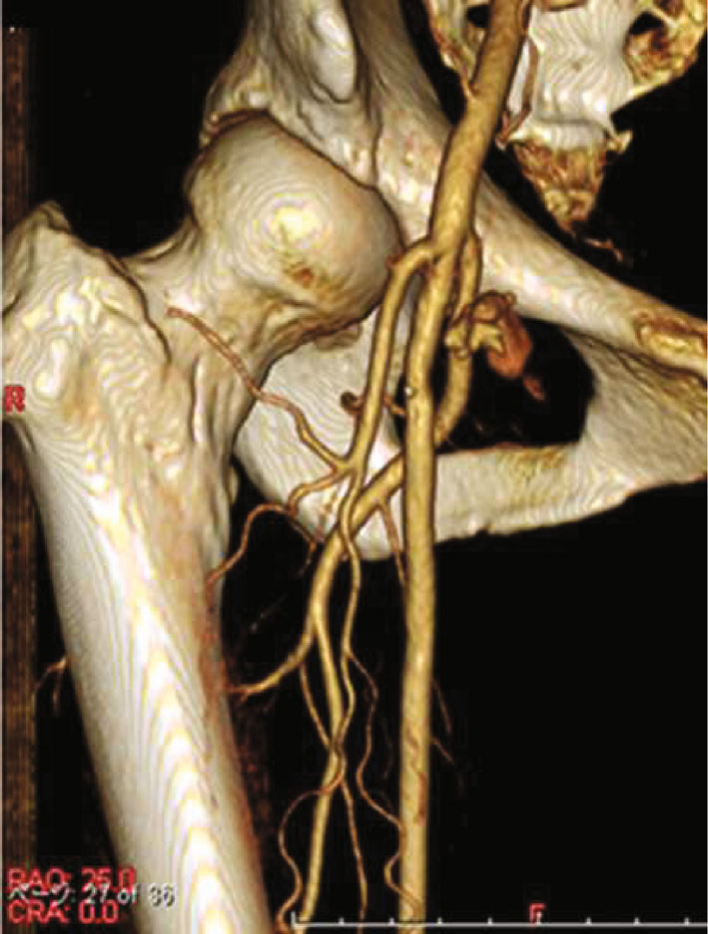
CT reveals a high femoral bifurcation and an aneurysm arising from the deep femoral artery.
